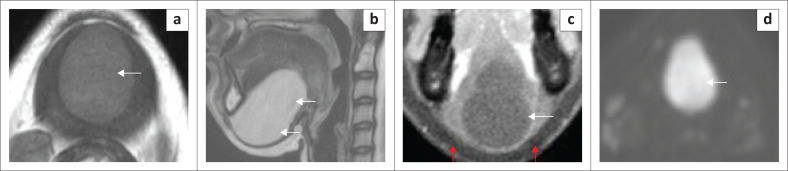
Epidermoid cyst in an adult female patient. T1 axial MRI neck with contrast (a) shows a large well defined, 5 cm, simple, non-enhancing cystic mass at the floor of mouth (a, white arrow) with intermediate homogeneous signal, splaying the anterior belly of the digastric muscles. T2 sagittal (b) shows homogeneous hyperintensity (white arrows). The contrast-enhanced T1 fat-saturated coronal image (c) shows a non-enhancing cyst (white arrow), limited inferiorly by an intact mylohyoid sling (red arrows). There is a marked restriction of diffusion on the high b-value diffusion-weighted image (d).
